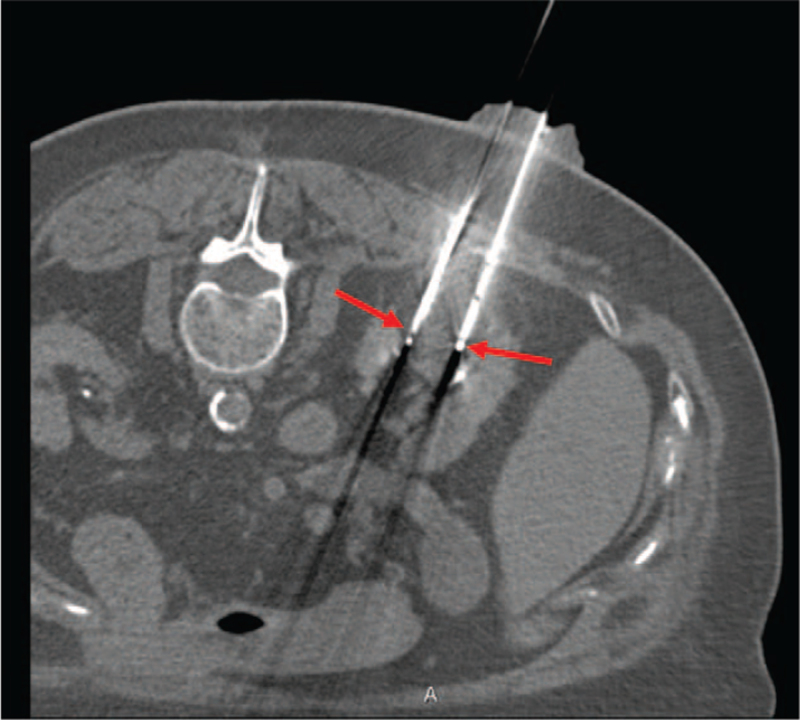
Intraoperative abdomen CT scan: the red arrows indicate two cryoprobes inserted into the target lesion.
